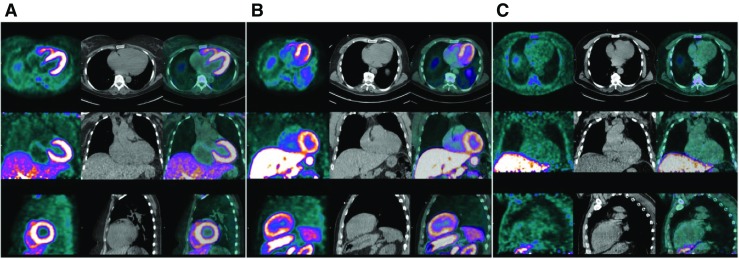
^18^F–florbetaben PET (left column in each panel), low-dose CT (middle column), and PET/CT images (right column) of representative AL patient (A), ATTR patient (B), and hypertensive control (C). ^18^F–florbetaben myocardial uptake is seen in patients with both AL and ATTR amyloidosis. W. Phillip Law et al. [50] J Nucl Med 2016;57:1733–1739
